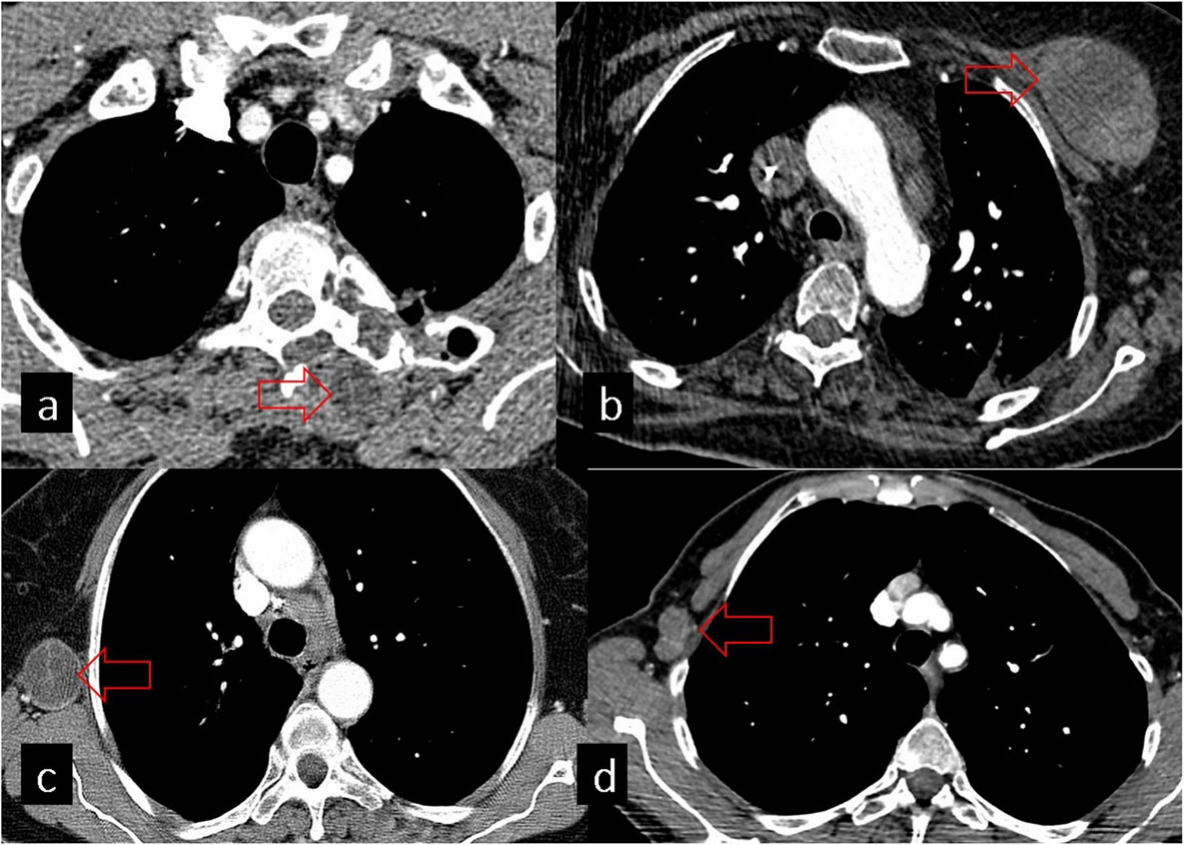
Hydatid cysts in thoracic soft tissues and differential diagnosis. a Axial CT image shows left paraspinal muscle involvement of HC (red arrow). b Pectoral hematoma as a differential diagnosis of HC seen on CT image (red arrow). c HC with daughter cysts located in the right axilla seen on the axial CT image (red arrow). d Necrotic lymphadenopathy secondary to lung cancer is seen in the right axilla
